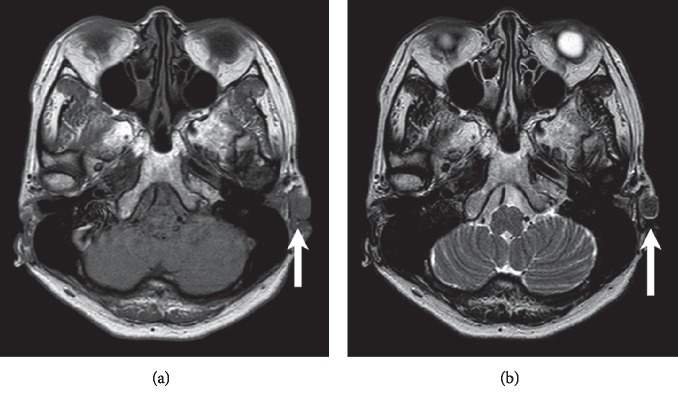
Magnetic resonance imaging revealing a solitary, well-circumscribed 11 × 10 mm tumor that was isointense on T1-weighted (a) and T2-weighted (b) images. There was no diffusion restriction on diffusion-weighted imaging (not shown).
